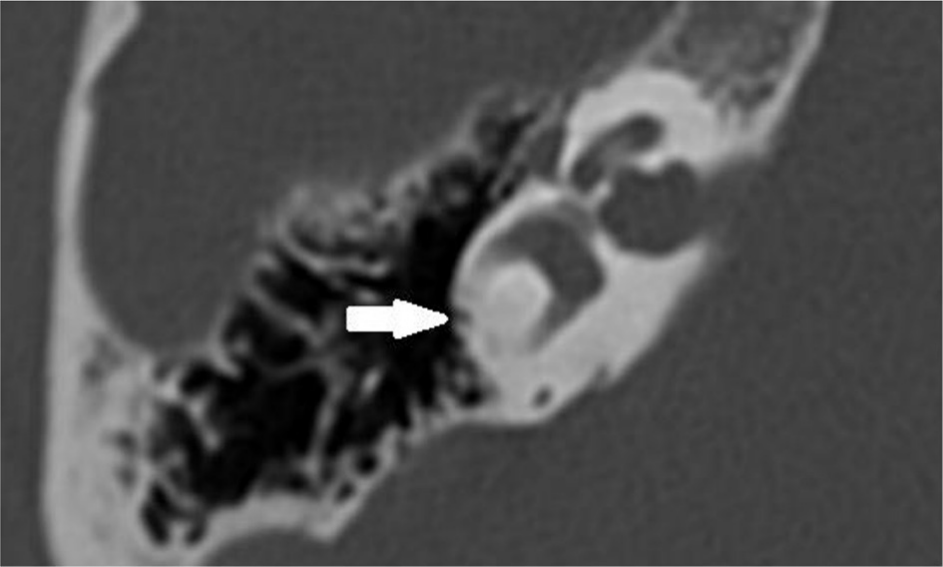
Axial temporal bone CT scan of the right labyrinth demonstrating narrowing and ossification of the lateral semicircular canal (arrow).
